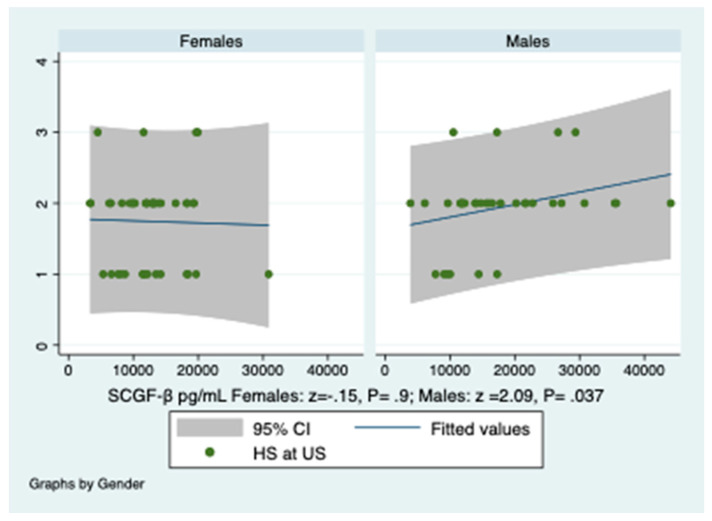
Prediction of the severity of hepatic steatosis at ultrasonography by SCGF-β levels. *Y*-axis reports the severity of hepatic steatosis. There is a gender-related difference of SCGF-β levels in predicting the severity of hepatic steatosis at ultrasonography, HS at US, expressed in grades (1–3). Ordered probit regression. A linear regression analysis was performed.
